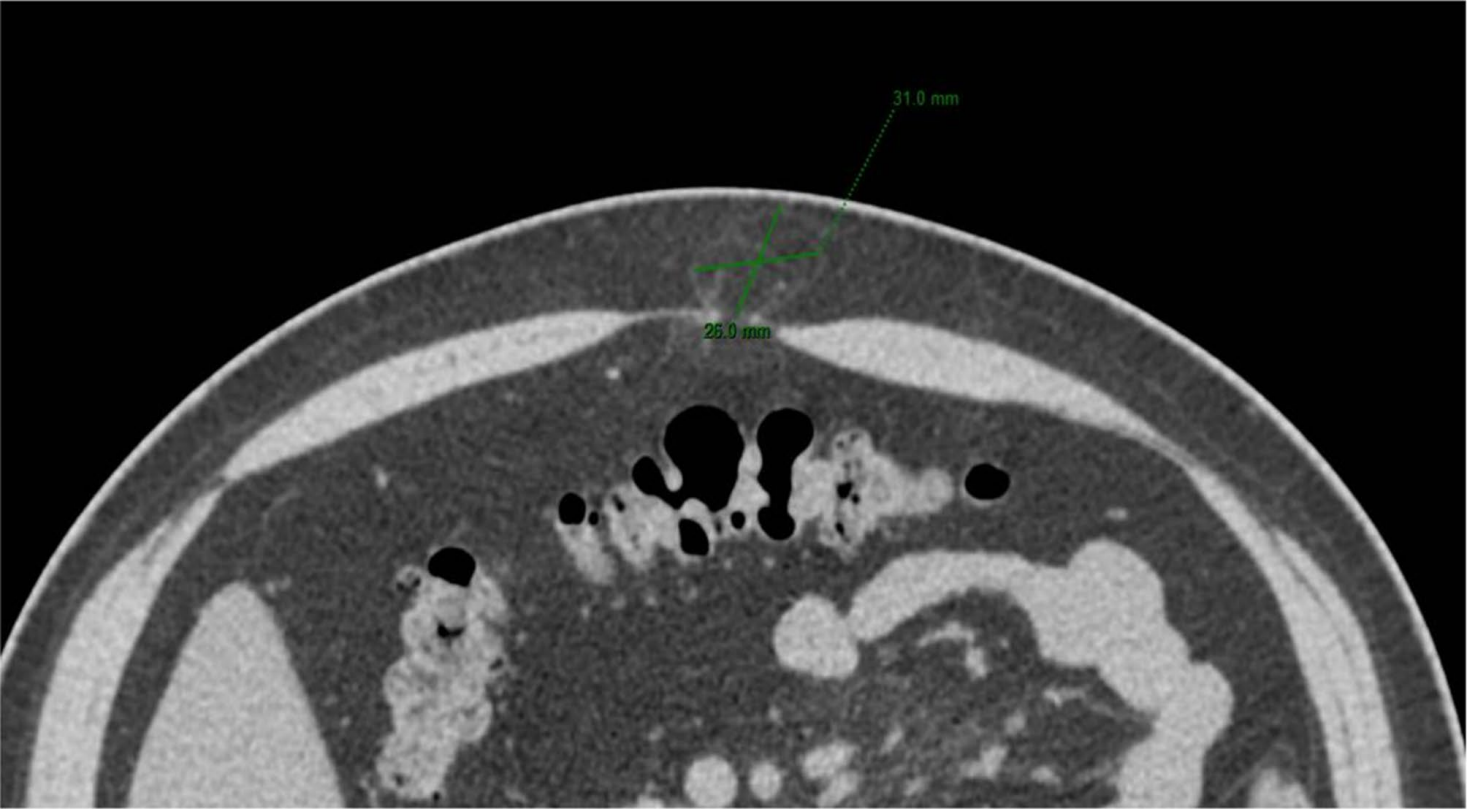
Preoperative computed tomography (CT).
